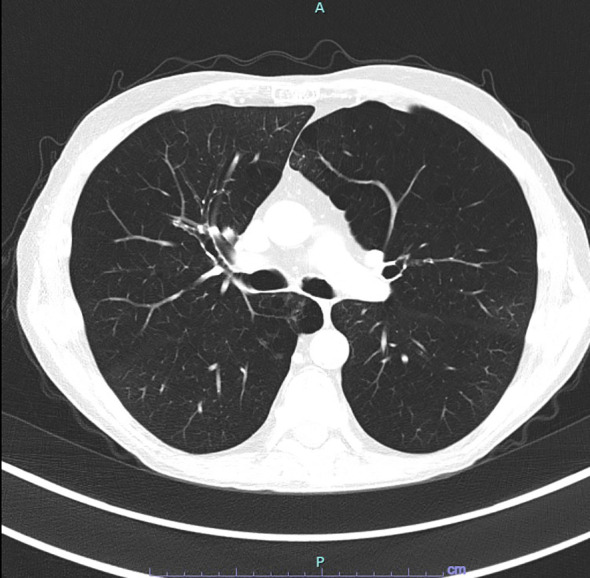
High resolution chest CT scan at baseline showing panlobular emphysema.
